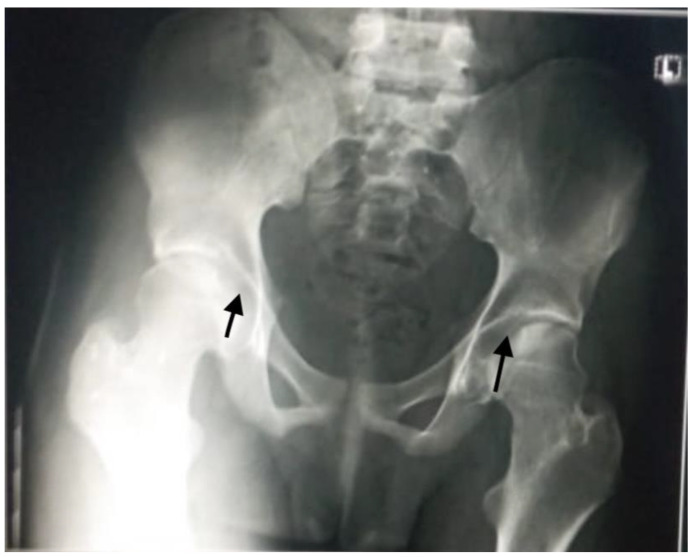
Plain radiograph showing avascular necrosis of the bilateral femoral head (black arrows). Commonly seen in sickle cell disease patients due to poor blood circulation from the disease condition.
